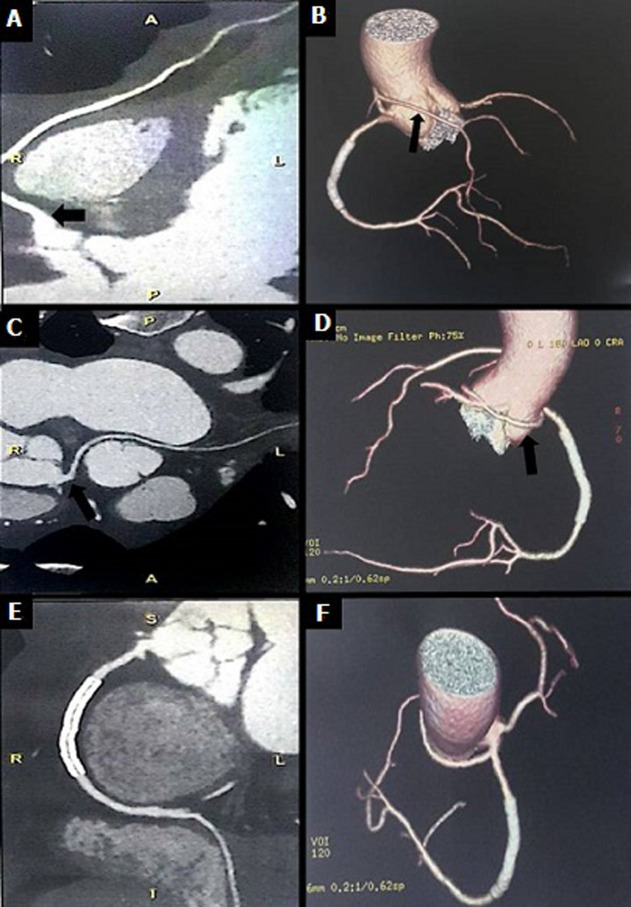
coronary computed tomography scan with 3D reconstruction showing: ectopic left anterior descending artery (black arrow) connected to the right sinus with a prepulmonary course (panel A and B); ectopic circumflex artery (black arrow) connected to the right coronary artery with a retroaortic course (Panel C and D), non ectopic right coronary artery with a permeable stent (Panel E and F)
